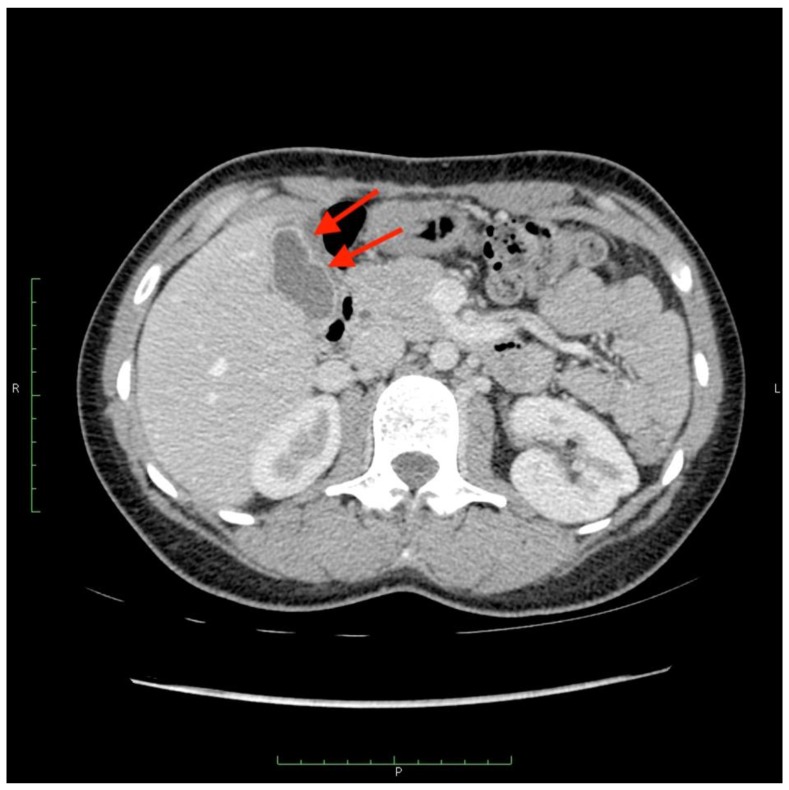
A computed tomography scan depicting asymptomatic cholecystitis (arrows) after the ^90^Y leak. The patient did not require surgery and was treated conservatively.
